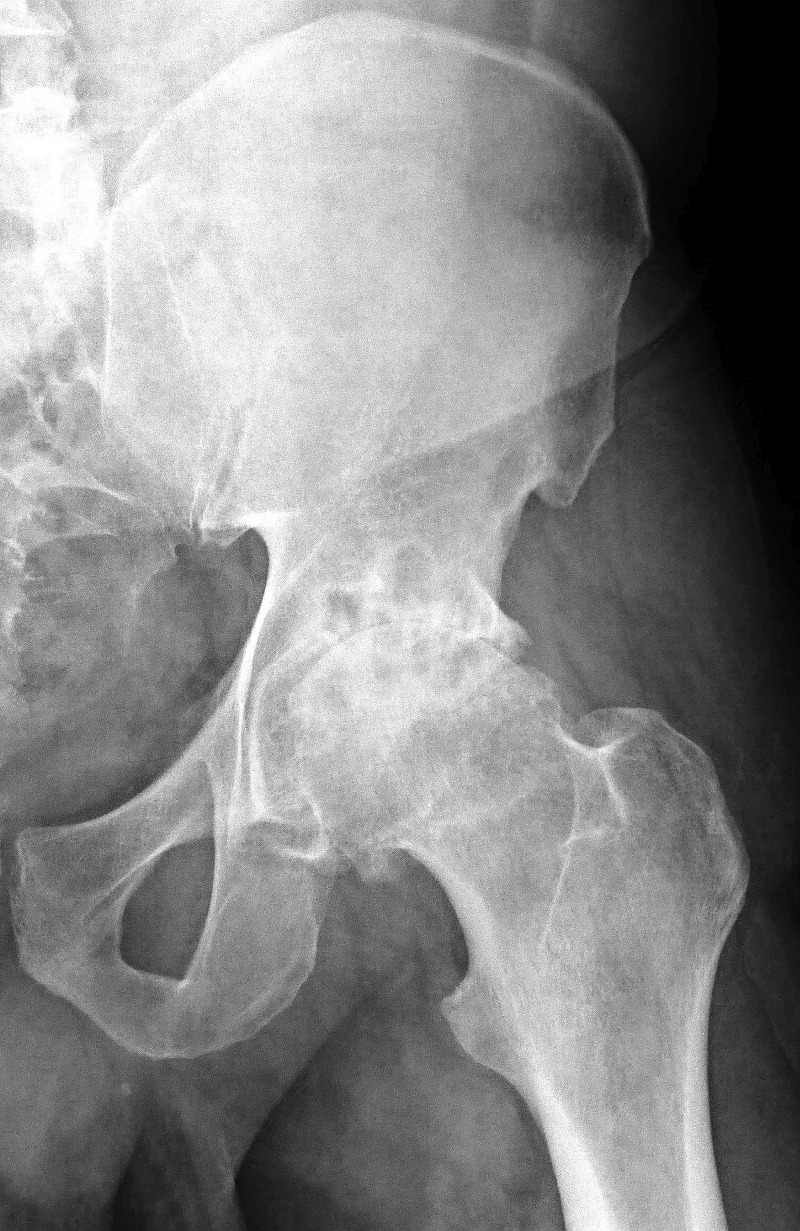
Plain preoperative radiograph (anteroposterior view) of the left hip showing arthritis of hip.
